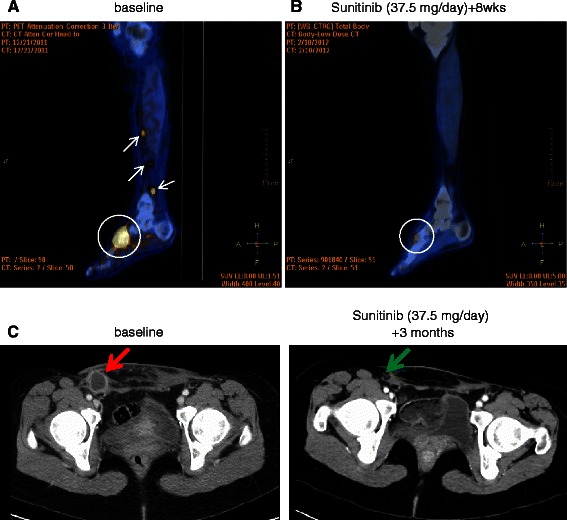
Response to sunitinib: FDG-PET and CT scan evaluation. (A) Baseline FDG PET/CT study: sagittal fused PET/CT image showing abnormal FDG uptakes in the left foot tumor (circle; SUVmax 12.0) and in soft tissue metastatic nodules in the ankle and leg (arrows); (B) After 8 weeks of treatment with SM 37.5 mg/day, PET/CT re-evaluation shows a complete metabolic response of the foot lesion (circle; SUVmax 2.5; ΔSUV −79%) and the disappearance of the soft tissue nodules. (C) CT scan (venous phase after contrast medium) shows a complete response to a right inguinal lymph node after 3 months of treatment with sunitinib (green arrow), compared to baseline (red arrow).
